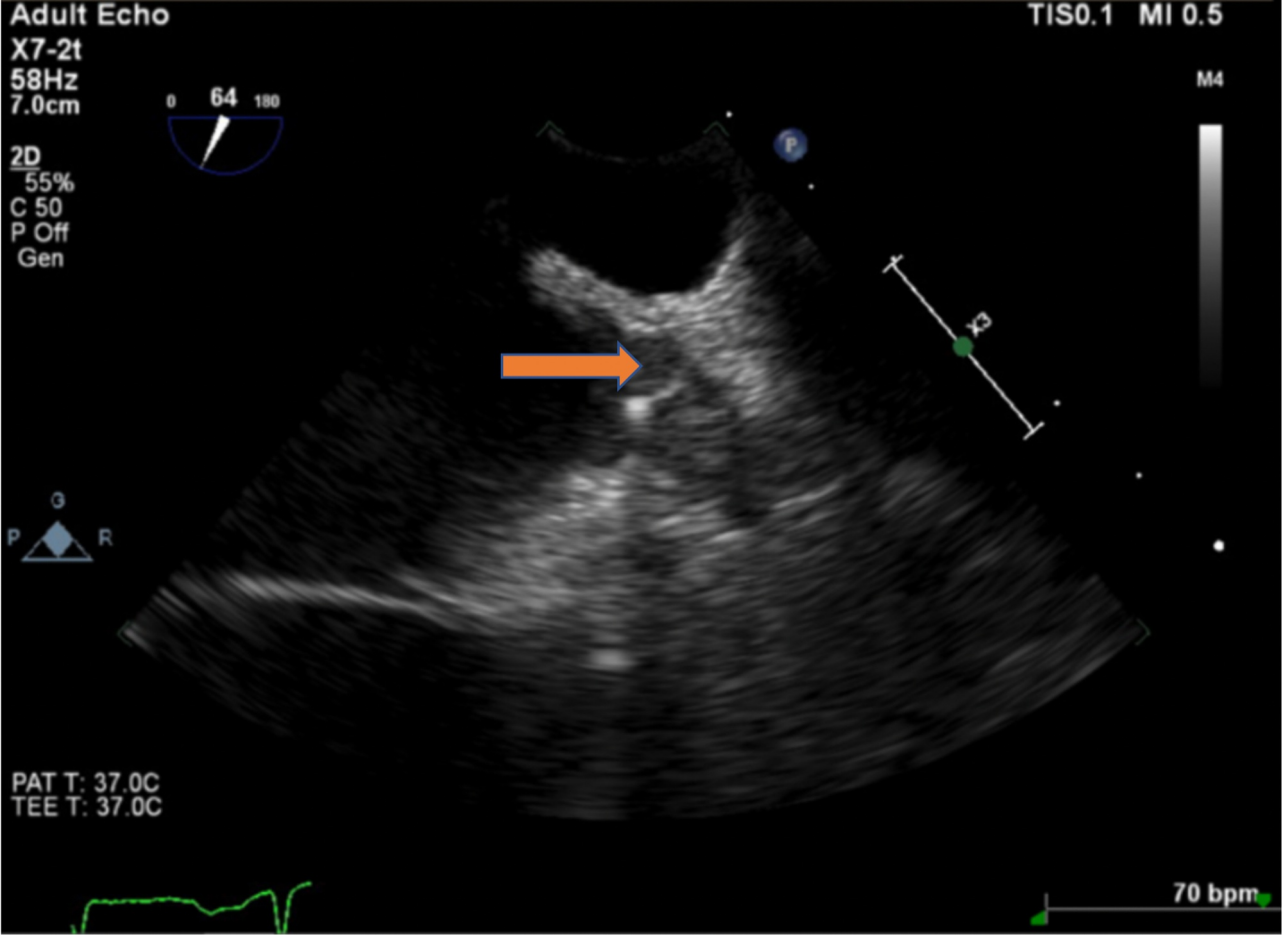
TEE six months after Watchman device implantation. The thrombus (red arrow) was grown back after cessation of anticoagulation. TEE, transesophageal echocardiogram
